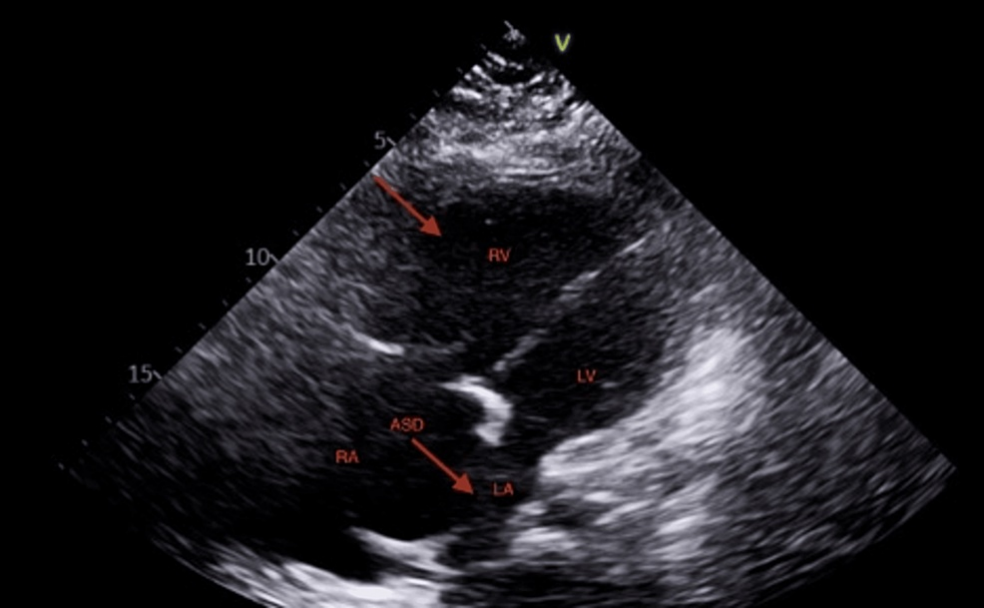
Transthoracic echocardiogram Right ventricle and atrial septal defect marked by red arrows.
